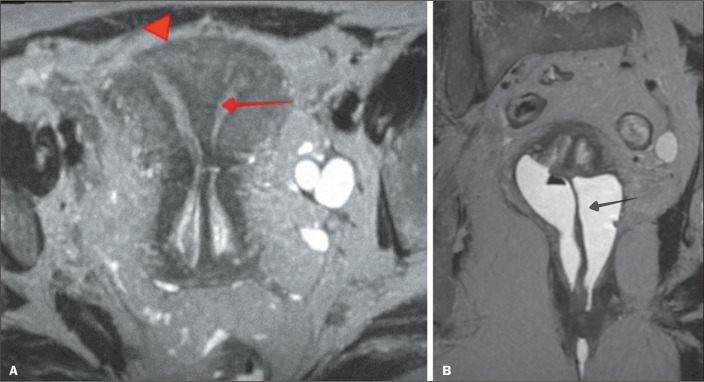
T2-weighted turbo spin-echo MRI scans, in the axial and coronal planes (A
